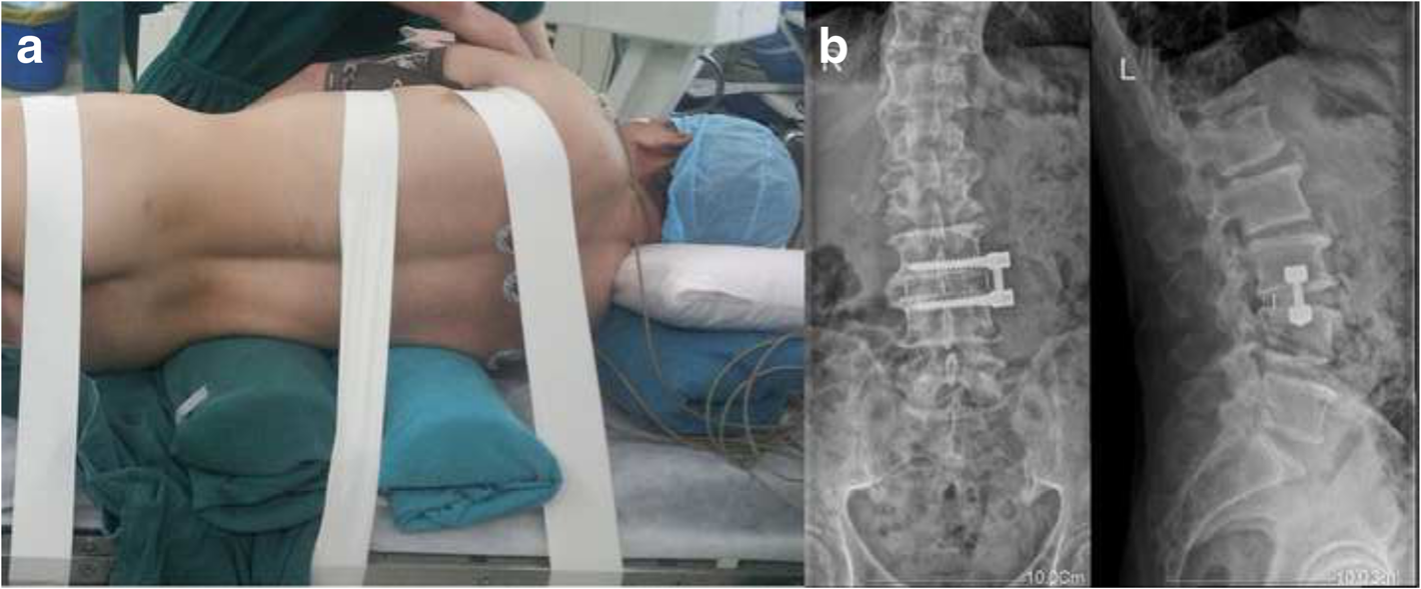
a Lateral incision. b Anterior-posterior and lateral X-ray image of DDD patients showing lateral trans-psoas interbody fusion at the L3-4 level with a side plate and interbody fusion mass as depicted by white markers
